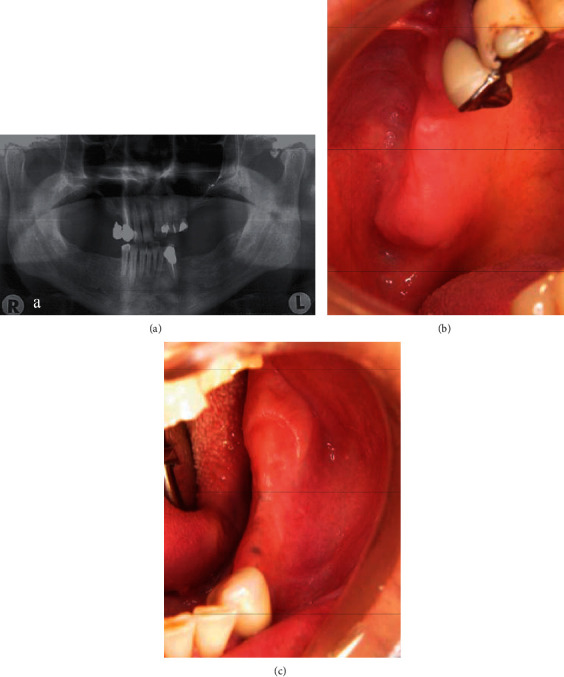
One-year follow-up after the end of RT treatment: (a) panoramic radiograph; (b) maxillary soft tissue healing; (c) mandibular soft tissue healing.
